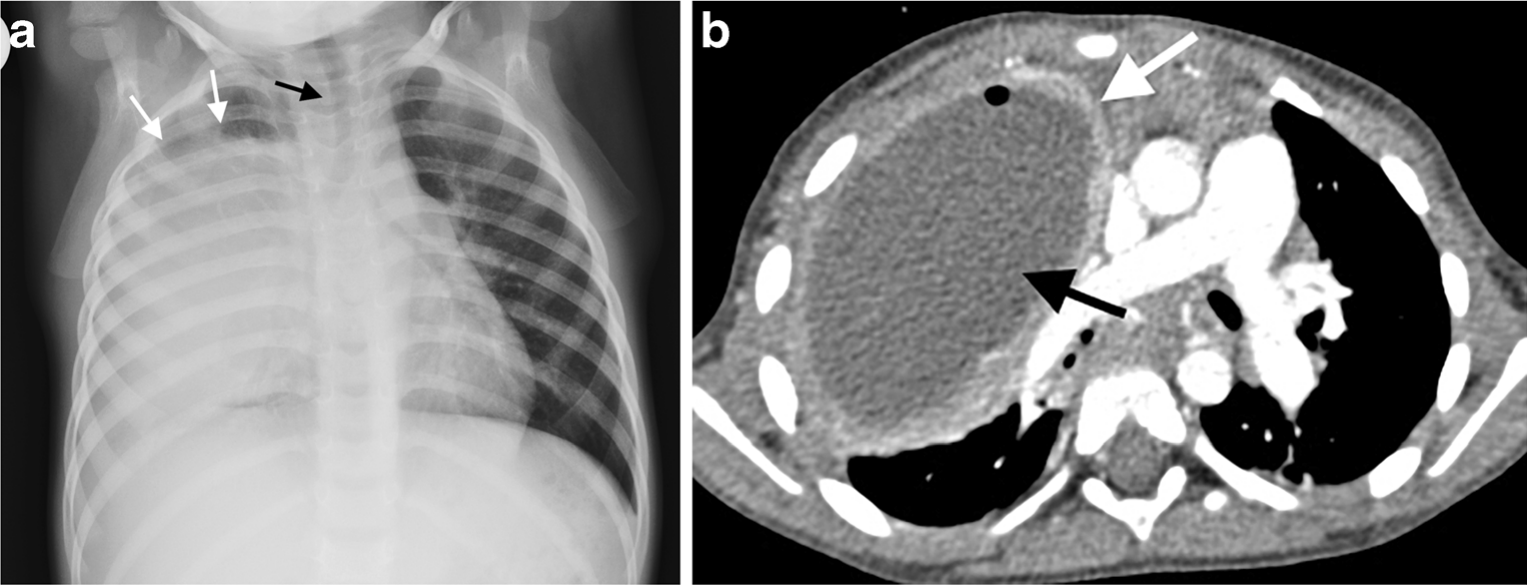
Lung abscess in a 2-year-old boy who failed to respond to antibiotic treatment for pneumonia. a Chest radiograph demonstrates an expansile dense opacity in the right lung with outwardly convex superior margin (*white arrows*) and mass effect on the mediastinum (*black arrow*). b Axial contrast-enhanced CT demonstrates a large abscess (*black arrow*) in the right lung with a well-defined, thick wall that shows some enhancement (*white arrow*) and displacement of the mediastinum to the left
